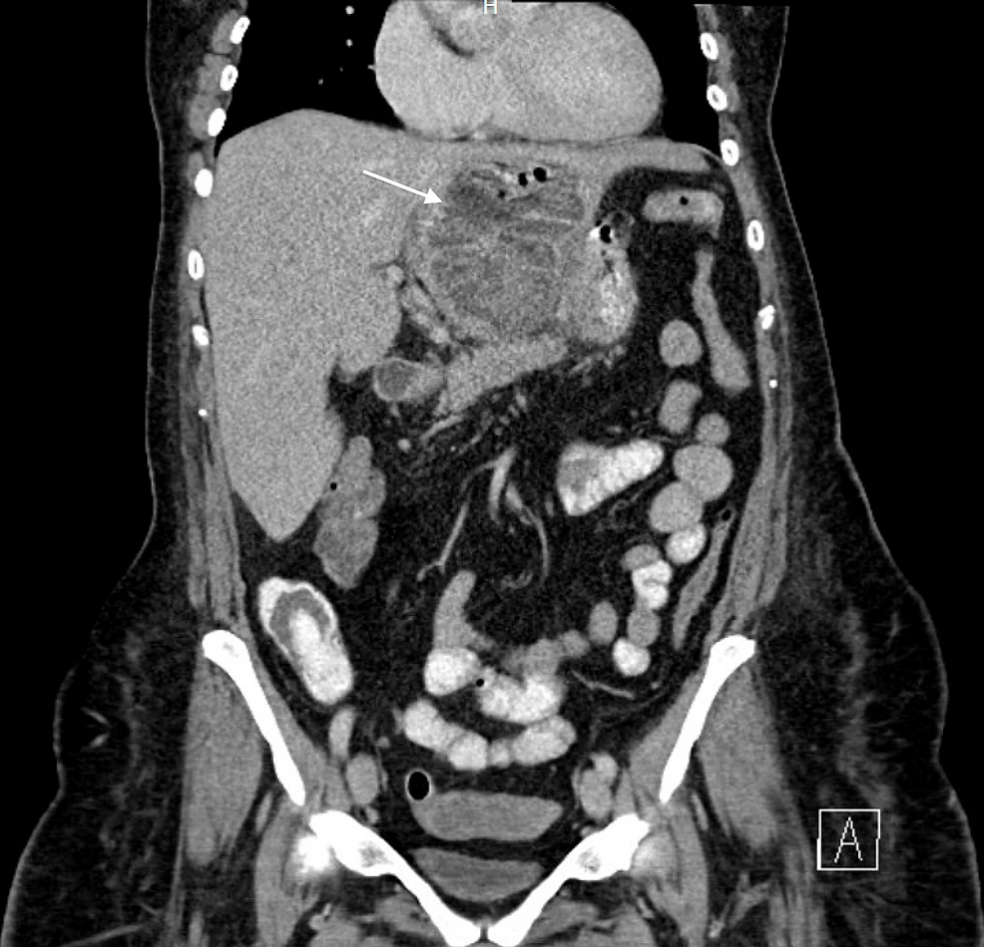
CT scan of the abdomen with intravenous contrast shows a liver abscess of the left lobe with multiple fluid levels on the coronal plane (white arrow)
